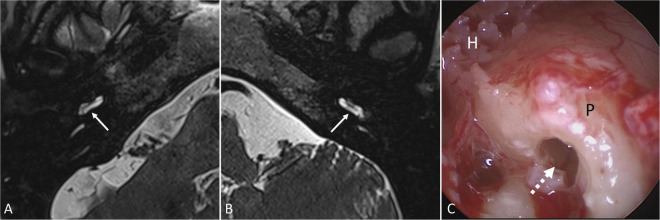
Magnetic resonance imaging (T2-weighted 3D-SPACE, axial, 0.4 mm) showing the basal cochlear turn of patient No. 17 (A) 6 months and of patient No. 16 (B) 9 months after intracochlear implantation of Ozurdex^®^ into the basal part of scala tympani. Regular fluid signals were found in the scala tympani of the basal turn (→) and in the entire inner ear without pathologic contrast enhancement and without signs of implant residuals (Department of Radiology, University Medicine Halle, Sabrina Kösling with permission). On cochlear implantation of patient No. 16, no signs of inflammation, foreign body reaction or residuals of the implant were found in the basal part of scala tympani (dashed arrow) (C). H: hypotympanon; P: promontory.
